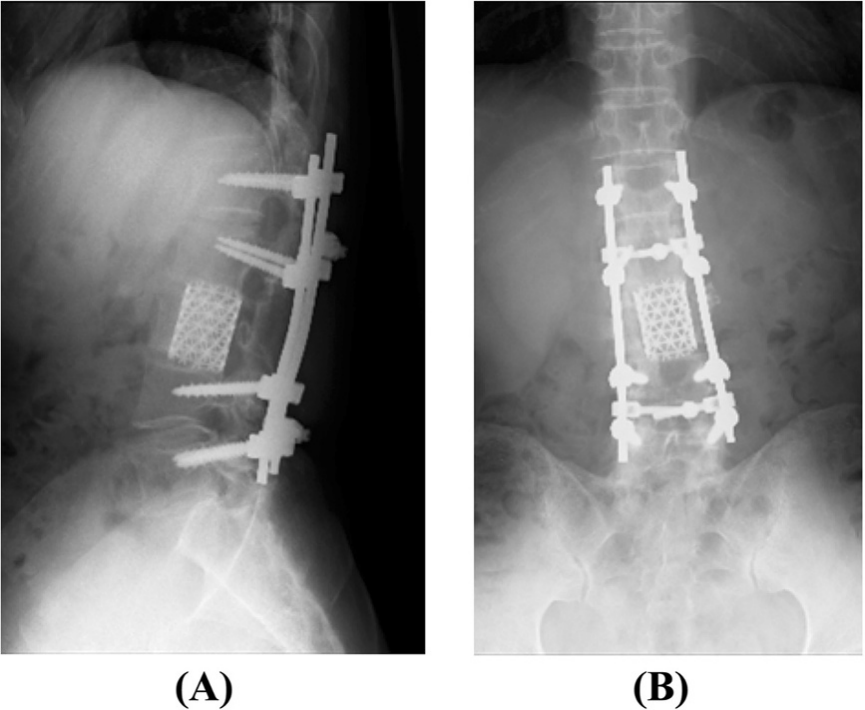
Post-operative lateral (A) and anteroposterior (B) X-ray of the lumbar spine. Circumferential fusion was performed using a titanium cage and pedicle screws.
